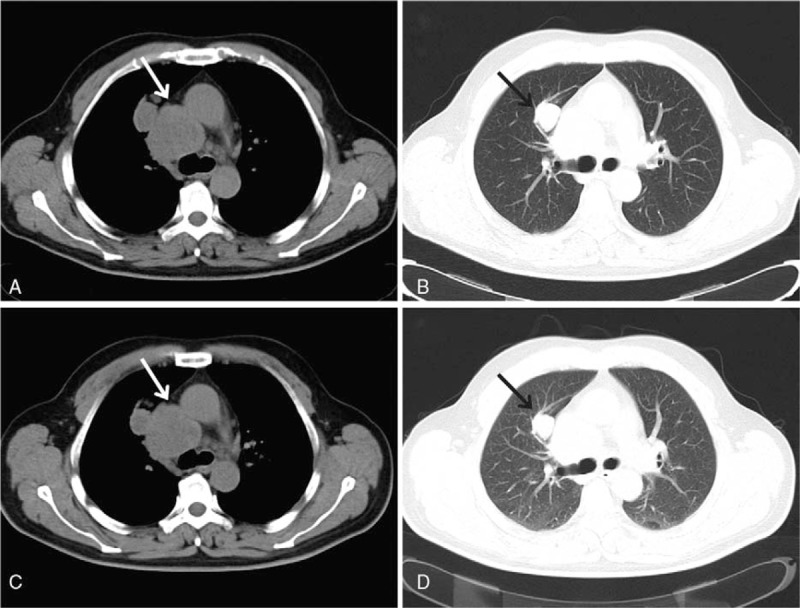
Pulmonary CT scanned that the right upper lobe nodules and mediastinal lymph nodes in 5 months (A, B) and 12 months (C, D) after surgery, respectively. CT = computed tomography.
